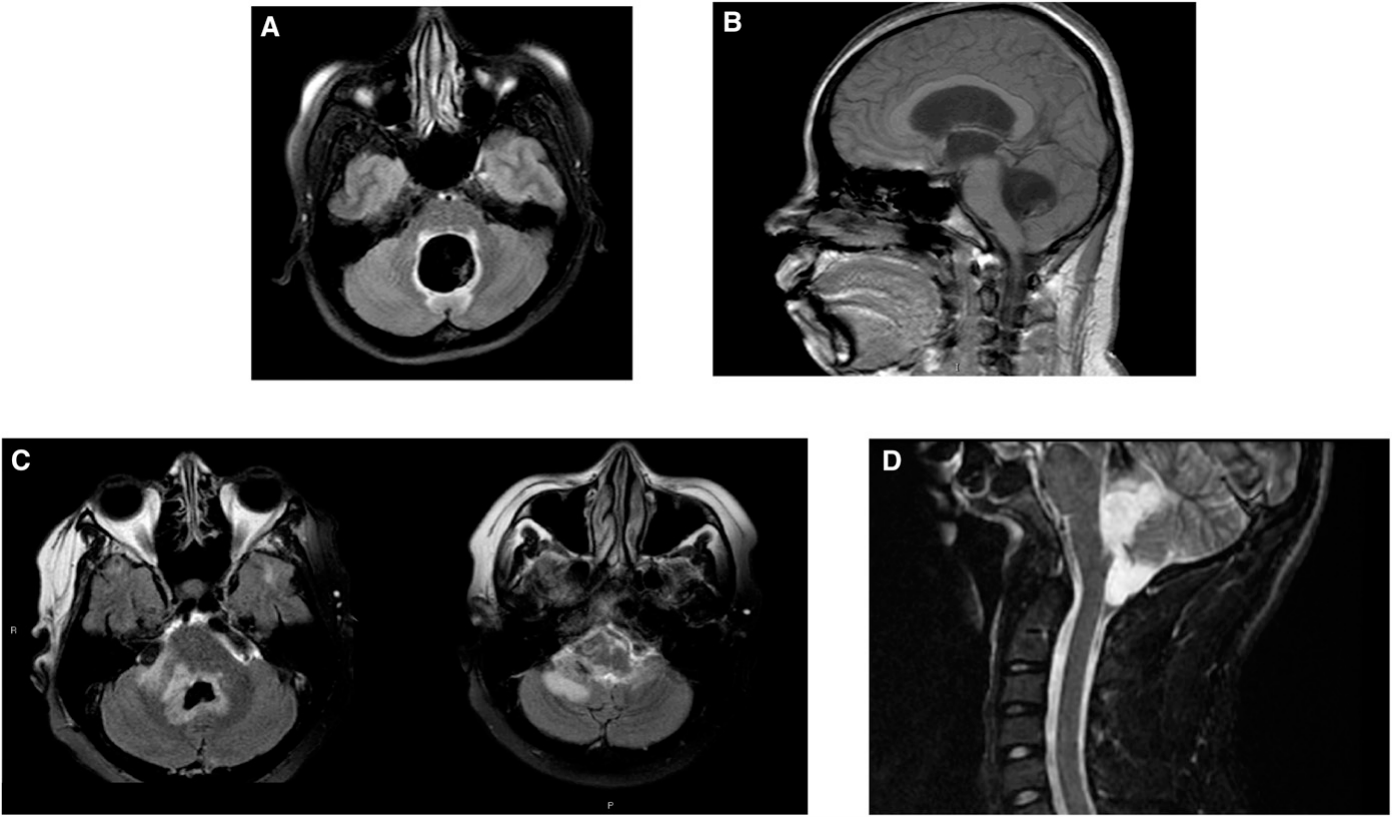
Panels (A) and (B) are Axial fast-attenuated inversion recovery (FLAIR) and sagittal T1W fast field Echo (FFE) imaging, respectively, of a patient with massive enlargement of the fourth ventricle with a scolex within the cyst. Hydrocephalus is apparent in the sagittal image. Panel (C) shows two FLAIR images demonstrating extensive periventricular edema around the fourth ventricle. Panel (D) is a lateral sagittal short-T1 inversion recovery image showing the fourth ventricle cyst that has exited the fourth ventricle into the cisterna magna by way of the foramen of Luschka.
